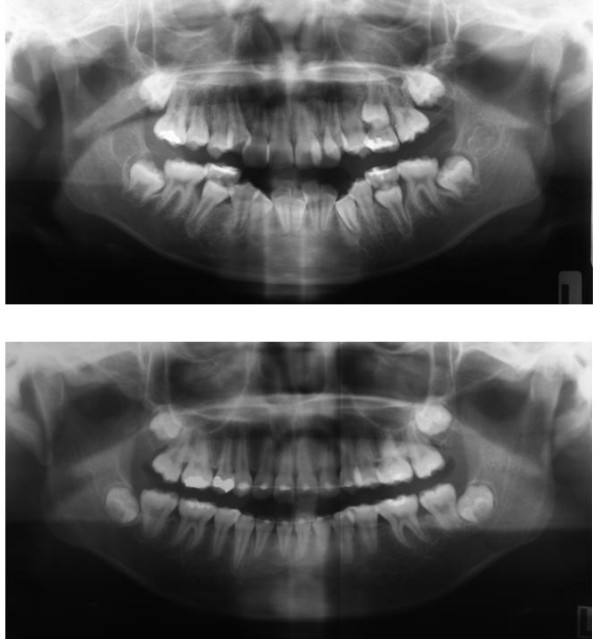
Post treatment panoramic radiographs. Post treatment panoramic radiograph showed reasonably root parallelism.
